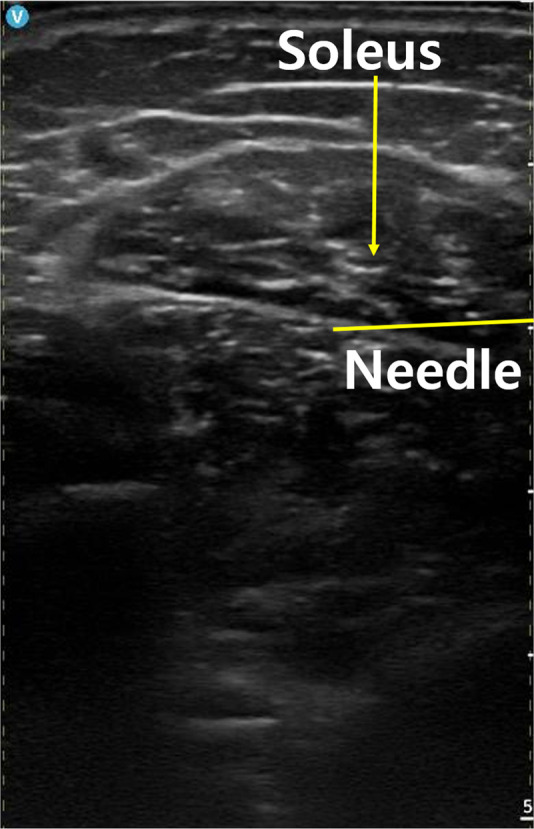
An ultrasound image depicting the cross-sectional view of the injection site.
